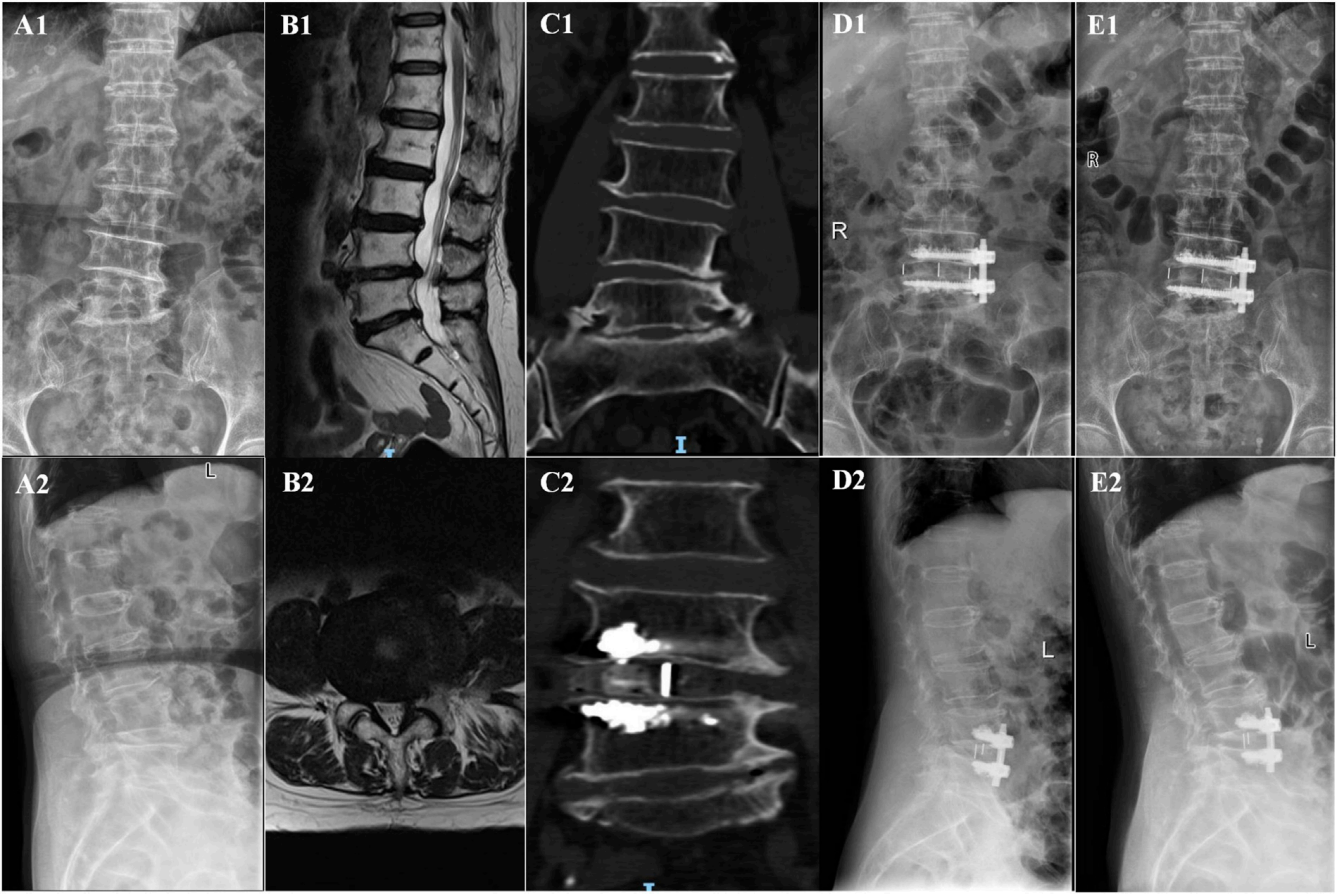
The radiological images of OLIF-BCAAS typical case. (A1,A2) Preoperative A−P, lateral X-ray film; (B1,B2) Preoperative sagittal and axial MR images; (C1,C2) Preoperative and postoperative coronal CT; (D1,D2) A−P and lateral X-ray film at 1 day postoperatively; (E1,E2) A−P, lateral X-ray film at last follow-up postoperatively.
